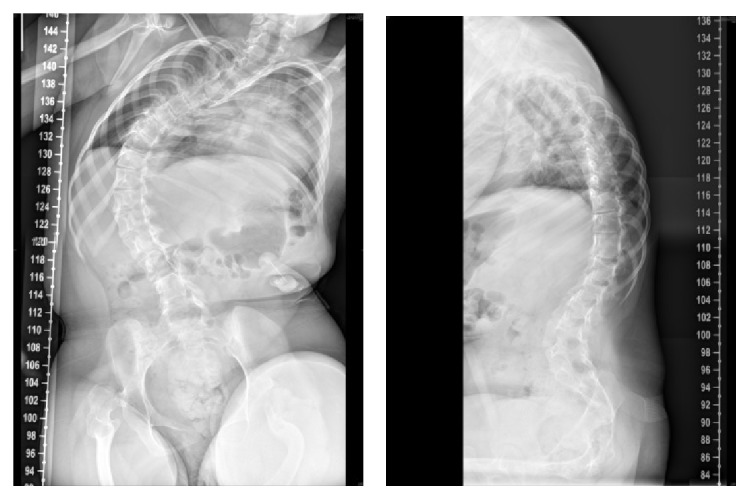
Preoperative radiographs showing a 100-degree scoliosis deformity in a severely involved cerebral palsy patient. The patient has pelvic obliquity of 15 degrees with significant coronal decompensation. Patient is nonambulatory with unilateral hip dislocation.
